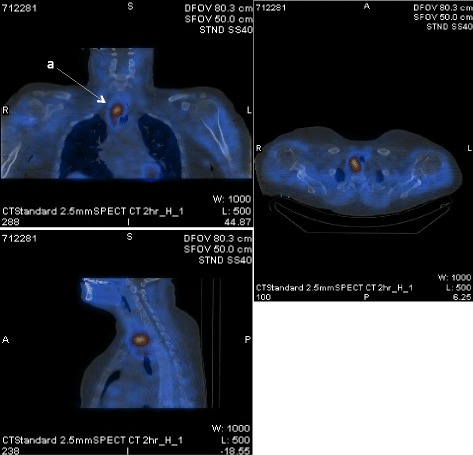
SPECT CT showing 3.7 cm soft tissue mass (a) in the right tracheoesophageal groove extending into the posterior upper mediastinum. There is some associated compression of the upper oesophagus which is slightly deviated to the left
